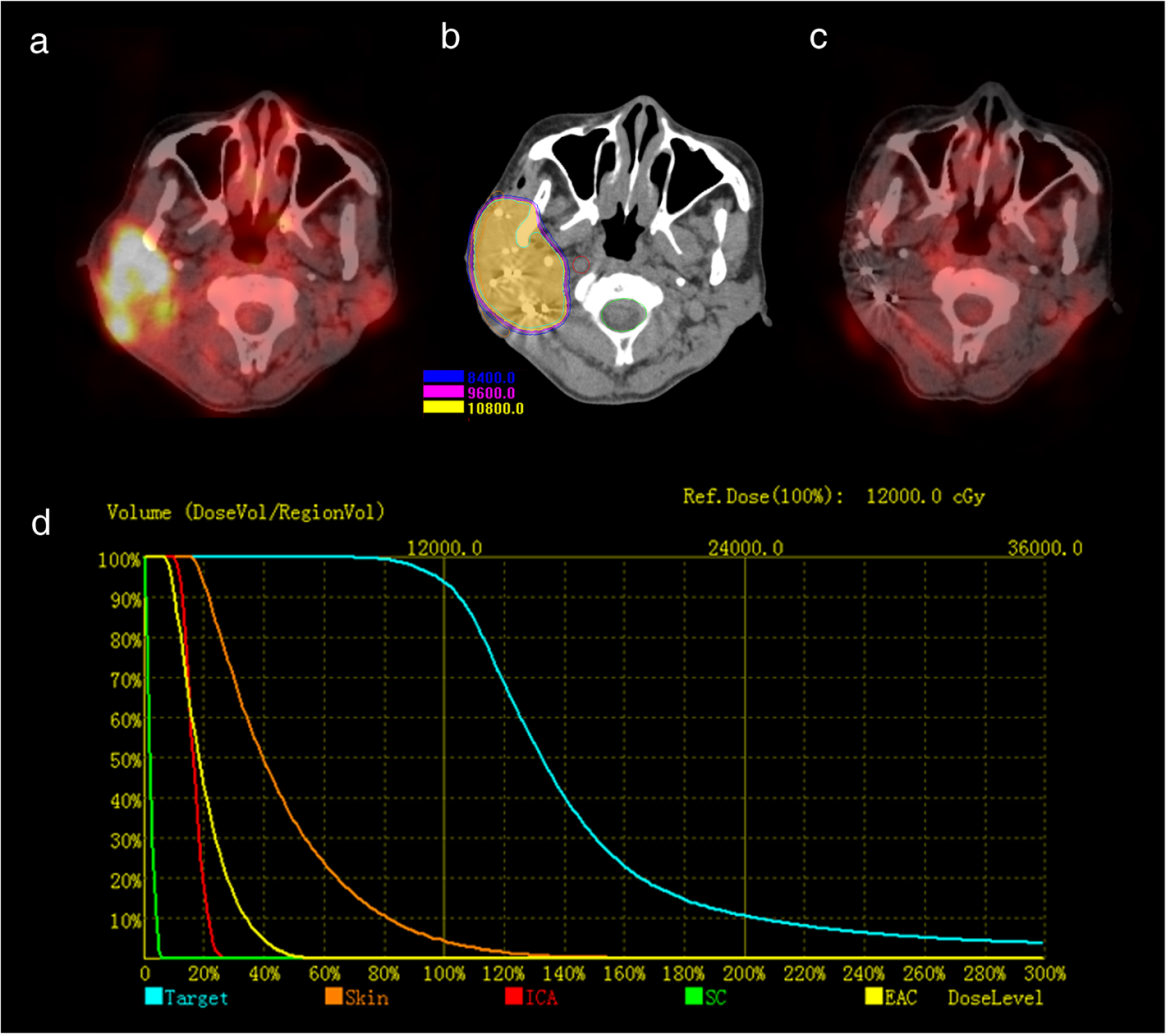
The procedure of brachytherapy based on PET/CT figure: Pretreatment PET/CT showed a T4 parotid gland carcinoma (a). Quality verification using postoperative CT images in the brachytherapy treatment planning system showed that the D90 curve covered the tumor area (b). PET/CT showed that the area of focal FDG uptake regressed 6 months after brachytherapy (c). Dose-volume histograms of the target area and organs at risk are shown (d). *ICA* internal carotid artery, *SC* spinal cord, *EAC* external auditory canal
